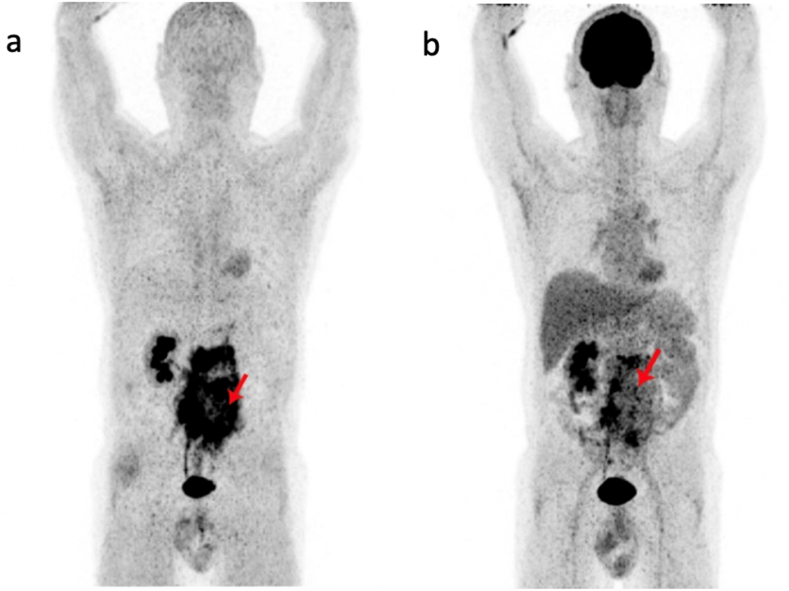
[68Ga]FAPI PET/CT (a) and 18FFDG PET/CT (b) images of restaging for urothelial carcinoma of the renal pelvis undergoing immunotherapy with pembrolizumab. Arrows indicate the retroperitoneal mass with high FAP-expression (left row) and intense, inhomogenous FDG-avidity (right row).
